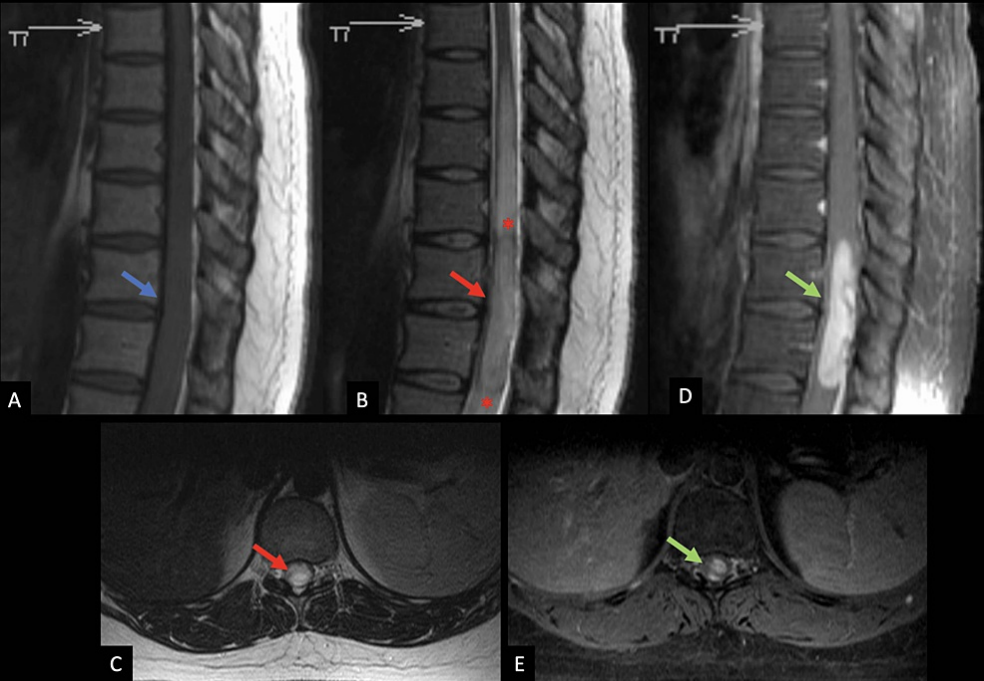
Preoperative MR imaging of the intramedullary mass in the lower thoracic cord An MRI of the thoracic spine from approximately mid-2020. Sagittal (A) the T1 weighted image (T1WI) shows an isointense signal mass in the lower thoracic cord at T11-T12 levels (blue arrow). The mass is more conspicuous and hyperintense in the signal on the sagittal (B) and axial (C) T2 weighted images (T2WIs) (red arrows). There is a subtle hypointense signal at its cranial and caudal margins (red asterisks). Extensive vasogenic edema is present from the T8 level to the conus (inferior extent not imaged). The mass demonstrates avid, mildly heterogenous contrast enhancement on sagittal (D) and axial (E) fat-suppressed, postcontrast T1WI (green arrows).
